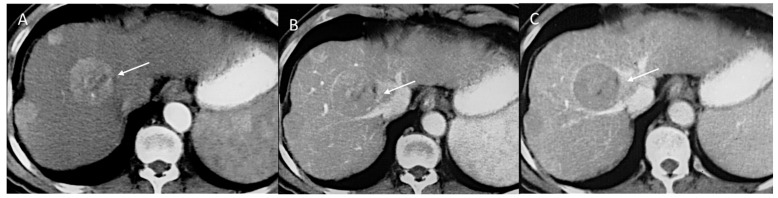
HCC on VIII seg. CT assessment. The lesion (arrow) shows APHE during arterial phase (A) of contrast study with washout in portal phase (B) and capsule appearance (C) in late phase.
